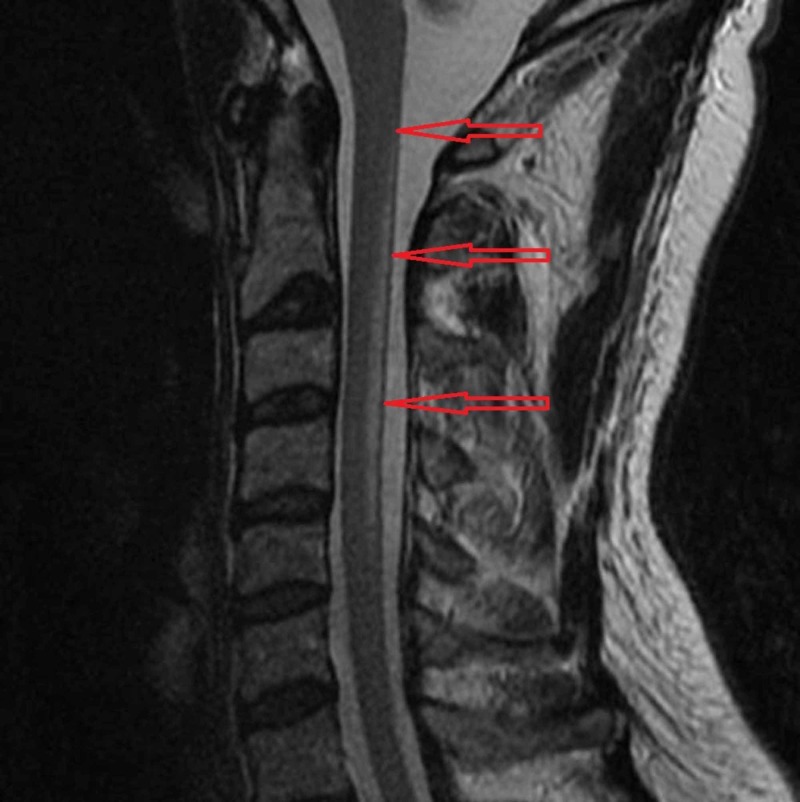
MRI reveals abnormal high T2 cervical cord signal within the dorsal columns extending from C2 to C5 highlighted by the red arrows The location of the signal abnormality is consistent with subacute combined degeneration of the cord. Image courtesy: [11]
